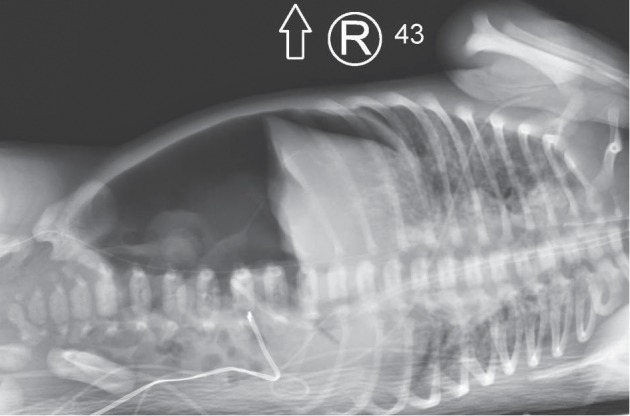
Lateral decubitus radiograph depicting a large pneumoperitoneum.
